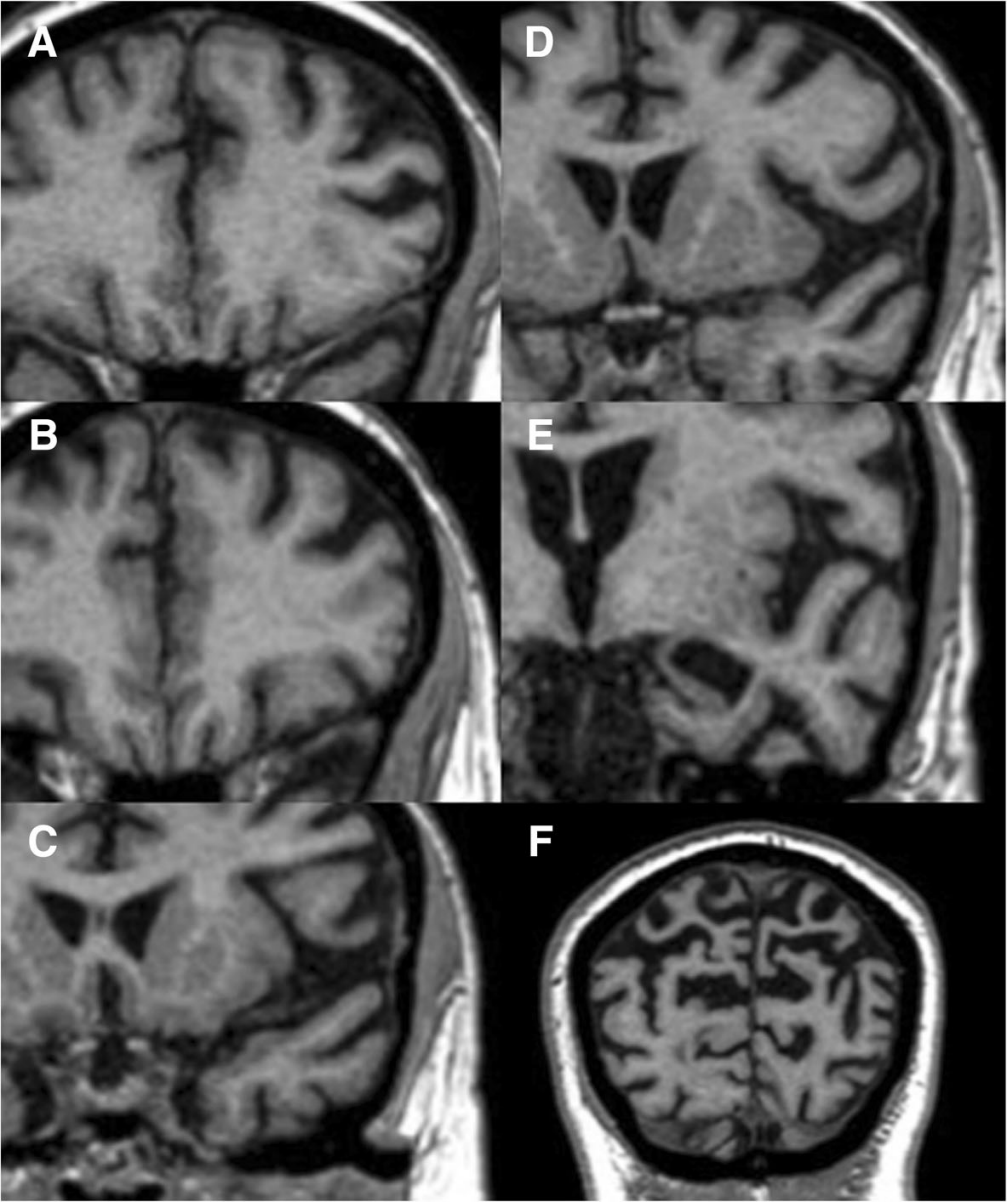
Coronal T1/3D reconstructions of a 48-year-old Down syndrome patient with dementia. a Grade 2 anterior cingulate gyrus atrophy (MRI visual rating scale). b Grade 2 orbito-frontal cortex volume loss. c Grade 2 anterior temporal cortex atrophy. d Grade 3 fronto-insular loss of volume. e Grade 4 medial temporal atrophy, including the amygdala and hippocampus. f Grade 3 posterior parieto-occipital cortex atrophy
